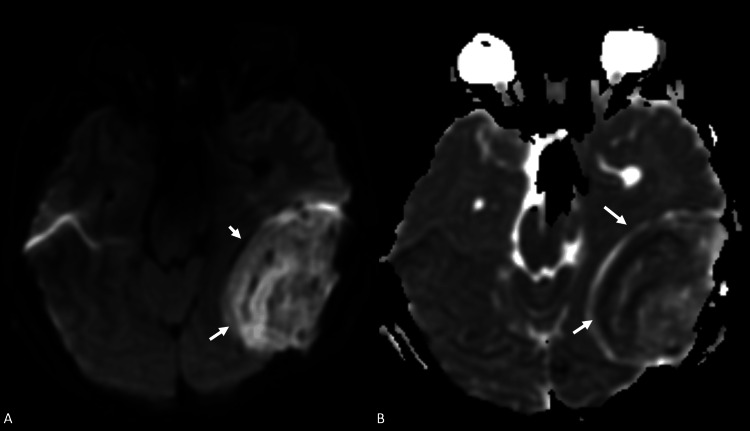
Preoperative DWI and ADC maps. DWI images (A) with the corresponding ADC map (B) demonstrate diffusion restriction within the lesion (white arrows). ADC, apparent diffusion coefficient; DWI, diffusion-weighted imaging
